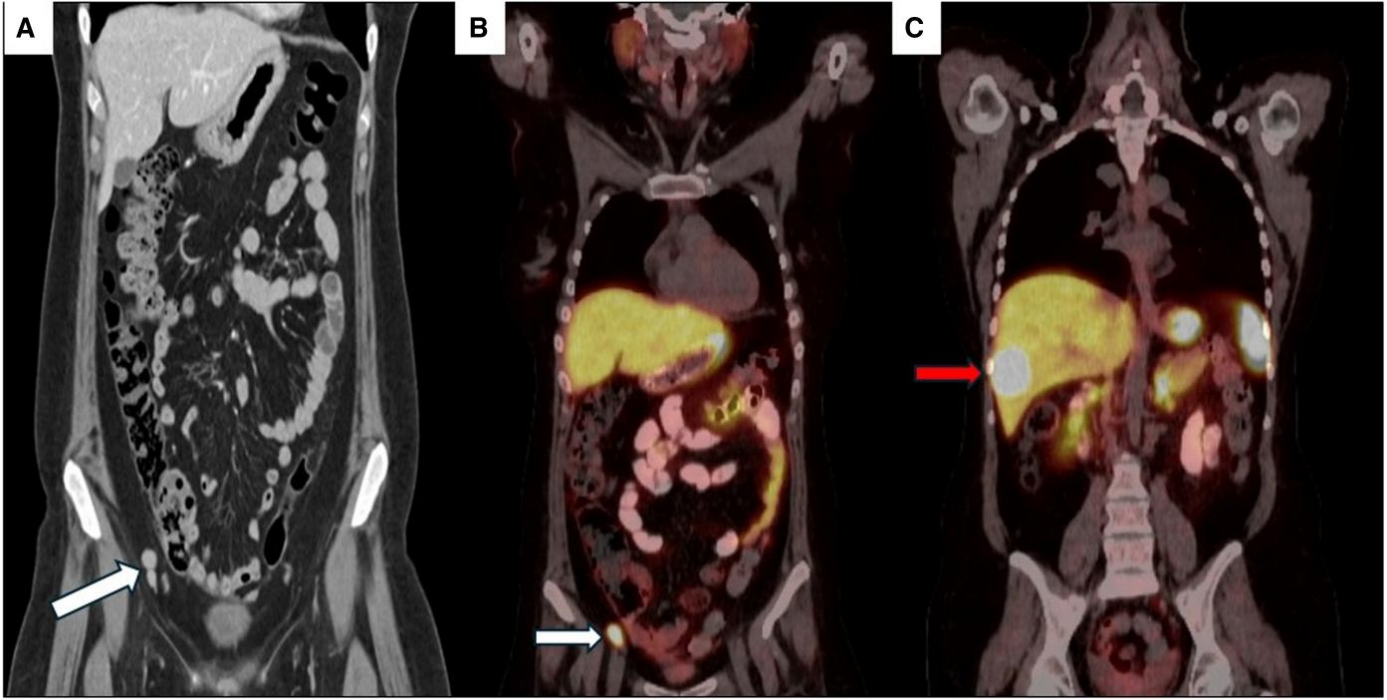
Metastatic neuroendocrine tumor imaging. (A) Coronal image from initial computed tomography of the abdomen and pelvis demonstrating a 3-cm appendiceal mass (arrow). (B and C) Coronal mages from Gallium-68 (Ga-68) DOTA-Tyr3-octreotate (DOTATATE) positron emission tomography/CT (PET/CT) redemonstrating appendiceal mass (B, arrow) and hepatic mass (C, arrow).
